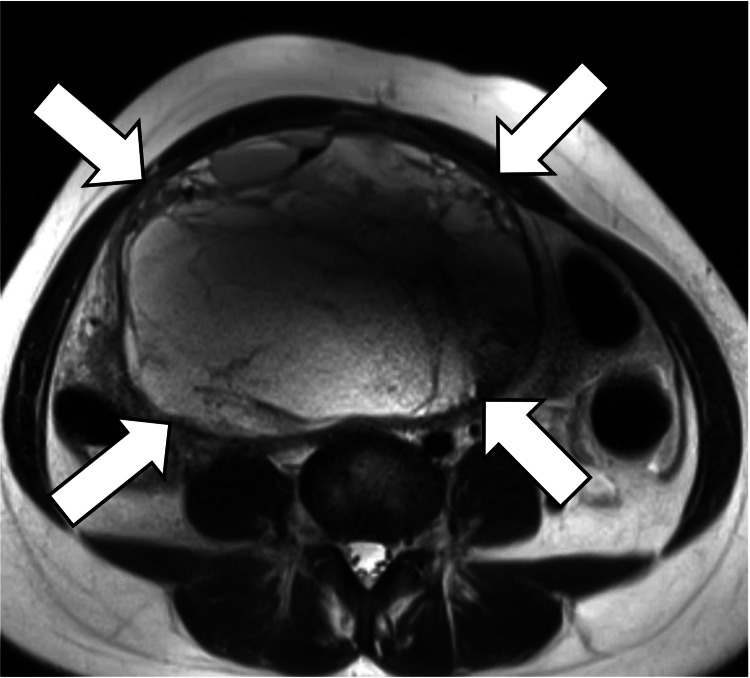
Axial, T2-weighted T2-weighted image shows a voluminous multilocular cystic mass (arrows) with several septations.
